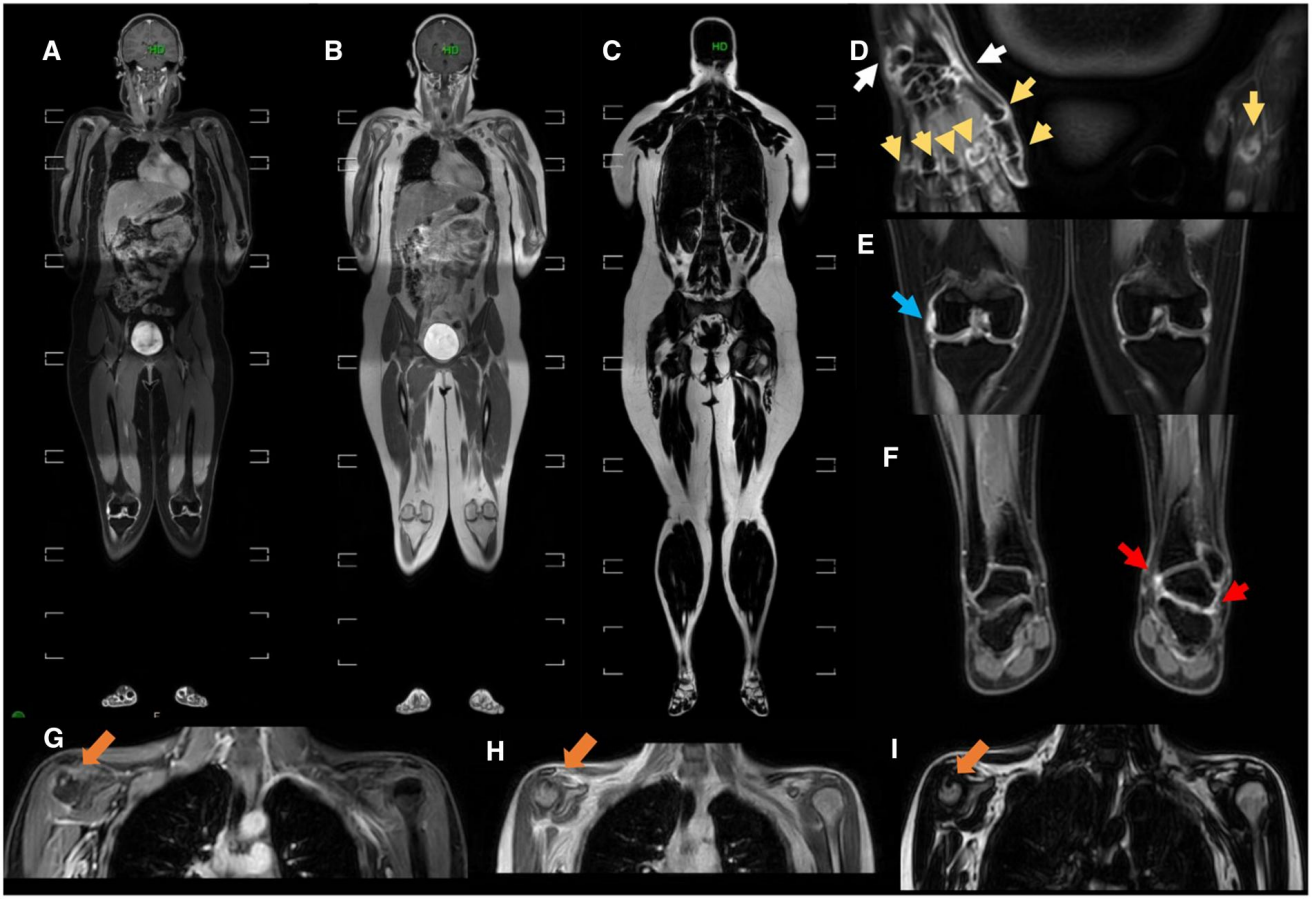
Post-contrast whole-body MRI images of two patients with polyarticular JIA (A-F and G-I). A. Whole-body water-only Dixon image. B. Whole-body in-phase Dixon image. C. Whole-body fat-only Dixon image. D. Grade 2 (G2) synovitis at the right wrist (white arrows), 1st–5th metacarpophalangeal joints and 1st interphalangeal joint and left 2nd metacarpophalangeal joints (yellow arrows). E. Right knee G2 synovitis (blue arrow). F. Left ankle and subtalar joint G2 synovitis (red arrows). G-I. Erosion (orange arrows) at right glenohumeral joint, demonstrating high signal on the water-only (G) and low signal on the in-phase (H) and fat-only images (I)
